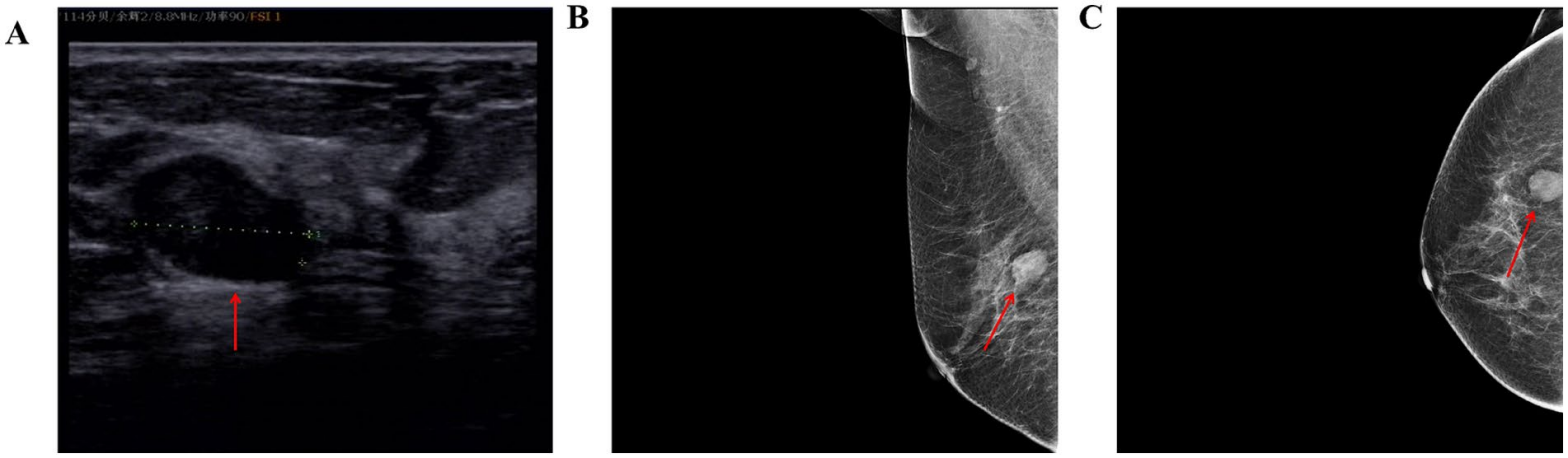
(A) Color Doppler ultrasound of the breast shows a 17.4 × 12.9 mm mass at the 9 o’clock position of the right breast, with a clear border and irregular shape. (B,C) Mammograms of the patient (red arrows representative lesion).
